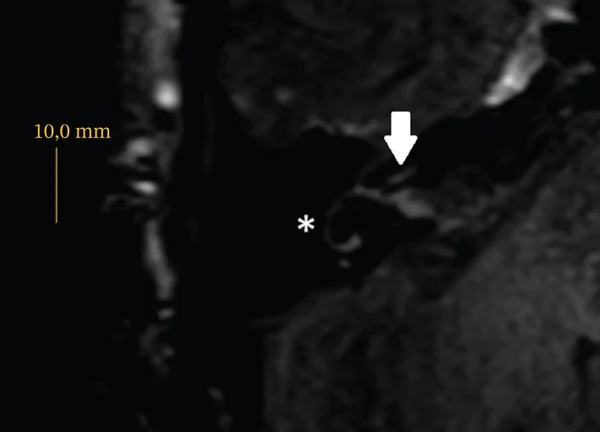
Axial slice of the 3D FLAIR sequence of the MRI of the same patient as in Figure 1 at the second time point with ELH Grade 2 in the vestibule (white star) and Grade 2 in the cochlea (white arrow) with a scale bar.
